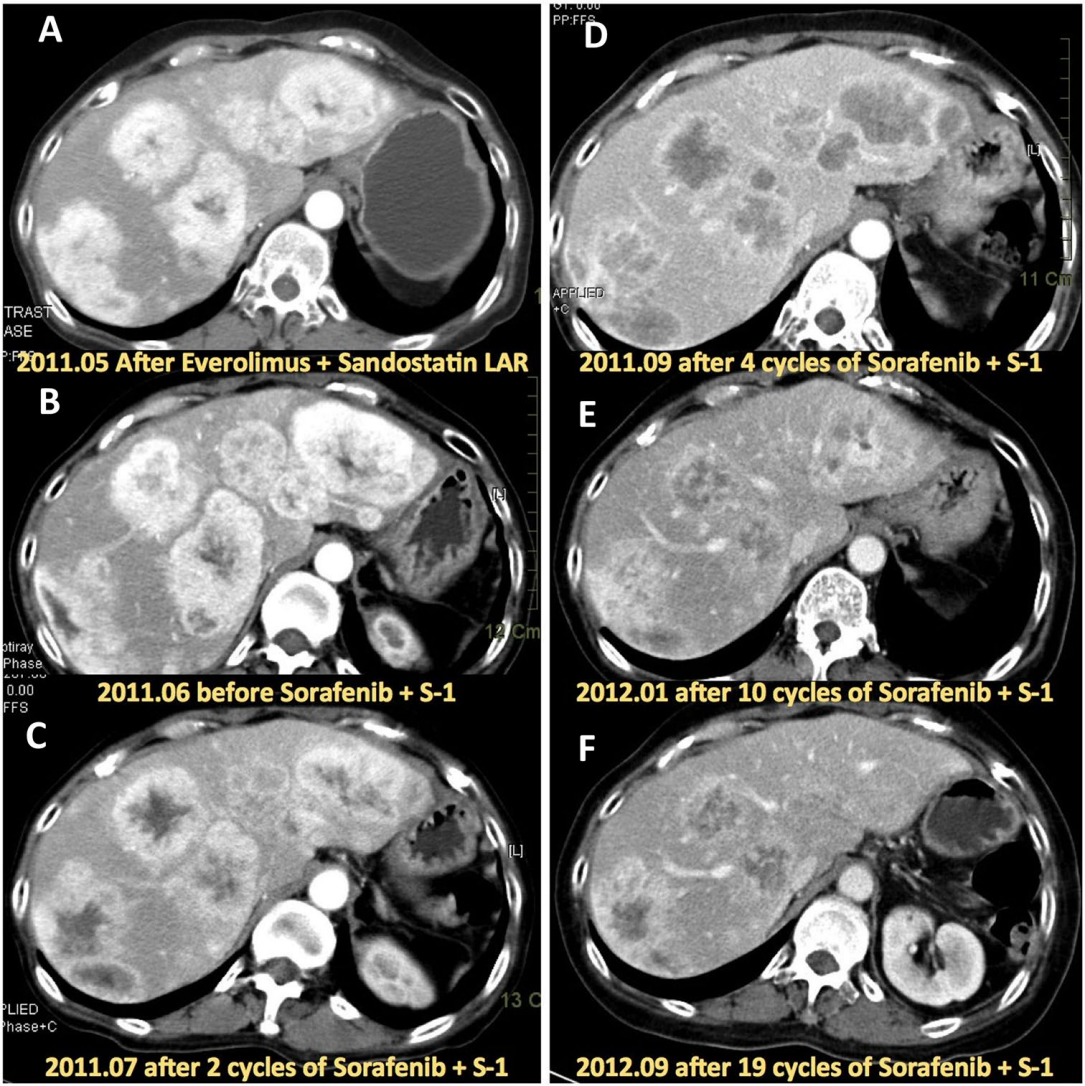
Serial CT images of a pNET patient enrolled in this study. (A) Hypervascular lesion of liver metastases; this patient failed to respond toeverolimus + Sandostatin LAR. (B) Rapid enlargement of liver metastases prior to treatment with sorafenib + S-1. (C)Regression of hypervascular lesion of liver metastases after 2 cycles of sorafenib + S-1. (D) Further regression of hypervascular lesion of liver metastases after 4 cycles of sorafenib + S-1. (E) Shrinkage of liver metastases after 10 cycles of sorafenib + S-1. (F) Persistent shrinkage of liver metastases after 19 cycles of sorafenib + S-1.
